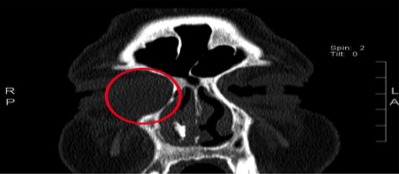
Preoperative coronal CT imaging of paranasal sinus showing dacryocystocele characterized by a low‐density, well‐defined cystic, fluid‐filled structure within the anteromedial aspect of the right orbit, adjacent to the nasolacrimal duct (circle).
